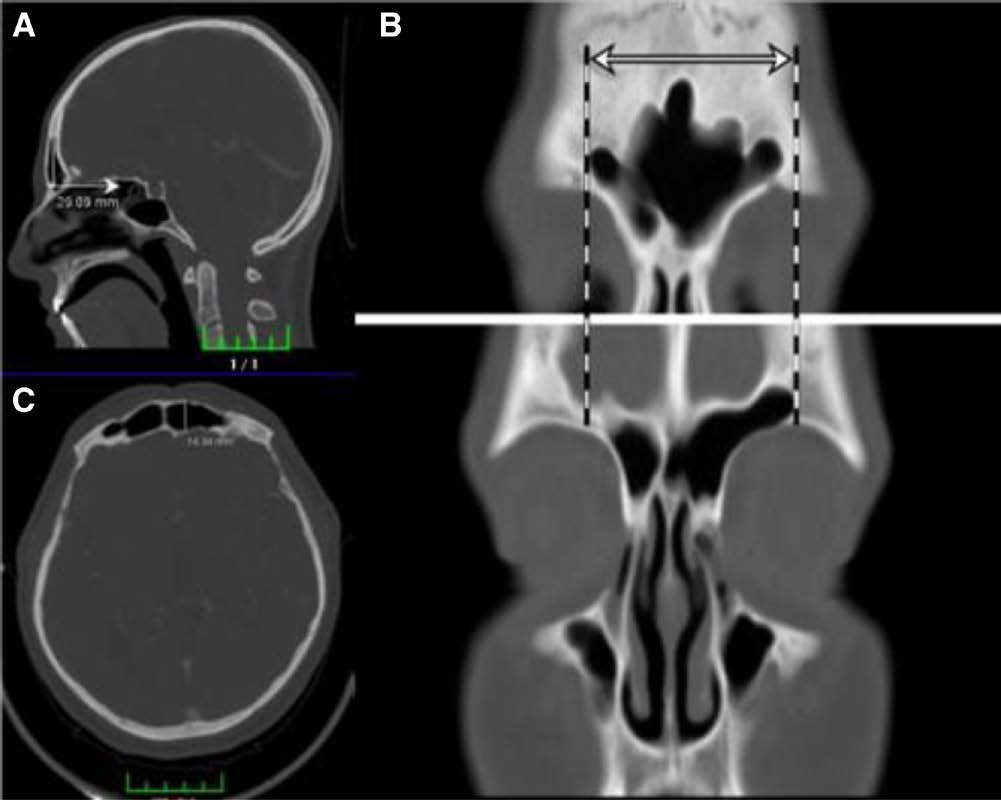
Demonstration of frontal sinus measurements on CT images. (A) Frontal sinus height (FSH), (B) frontal sinus width (FSW), (C) frontal sinus depth (FSD). CT = computed tomography.
